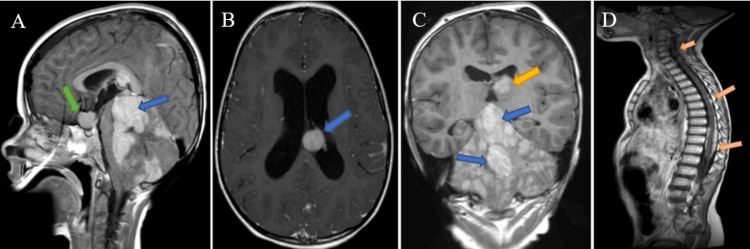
Contrast-enhanced T1 MRI Multifocal lesions of the cerebellum (blue arrow) and suprasellar region (green arrow) (A), LLV (B), left thalamus (yellow arrow), cerebellum and fourth ventricle (blue arrows) (C), and all levels of the spinal cord (D). MRI of the spinal cord was performed after endoscopic biopsy. LLV, left lateral ventricle
